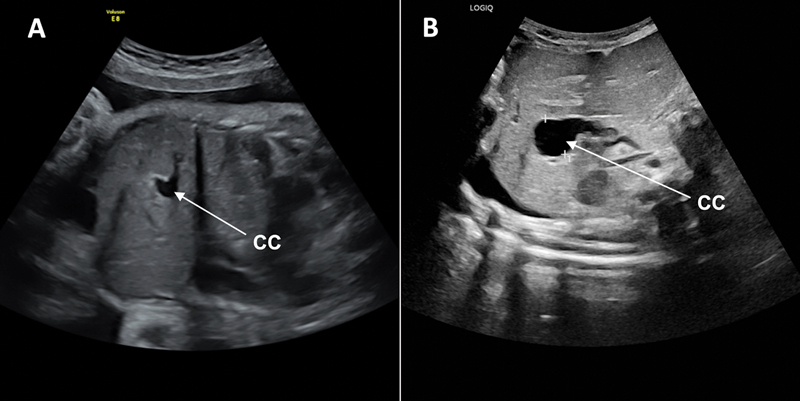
Ultrasound (US) imaging showing the intrahepatic biliary structure originally identified as the gallbladder, but ultimately found to be the choledochal cyst (CC). (
